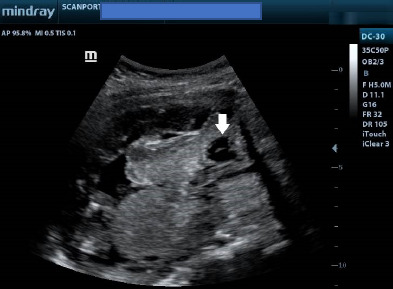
Ultrasound image of the lower uterine segment showing the placenta completely covering the internal cervical os. Note also the cystic lesion (white arrow) at the anterior part of the placenta, in keeping with a placental cyst
